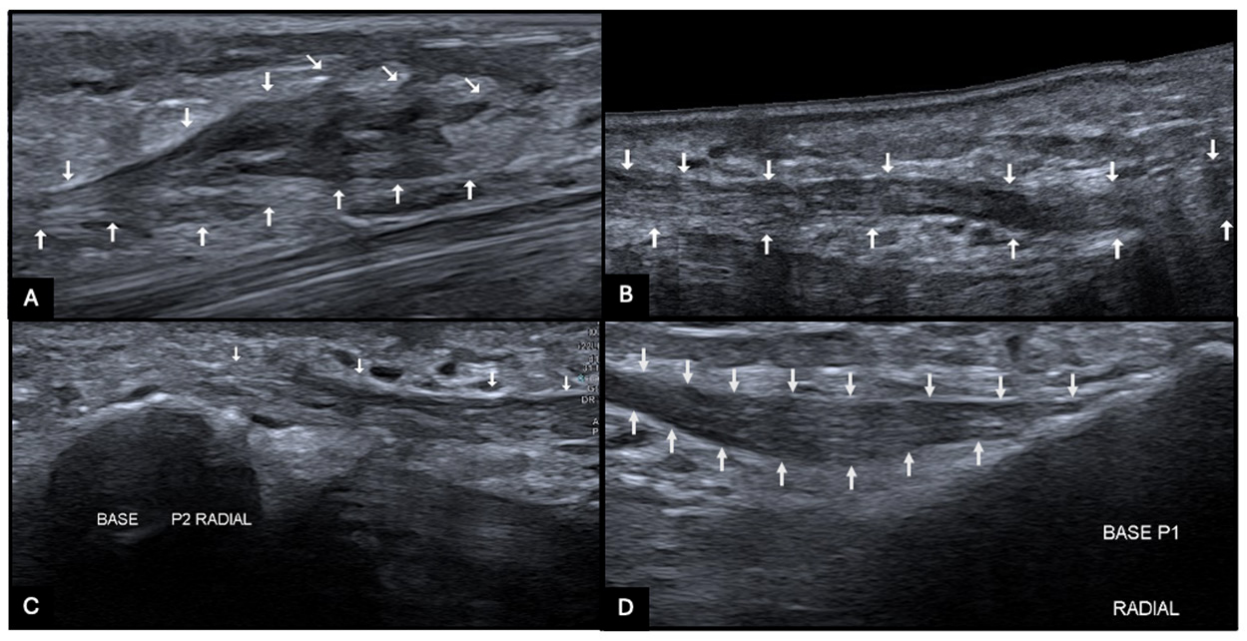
Ultrasound showing a superficial hyperechoic digital cord (arrows).
